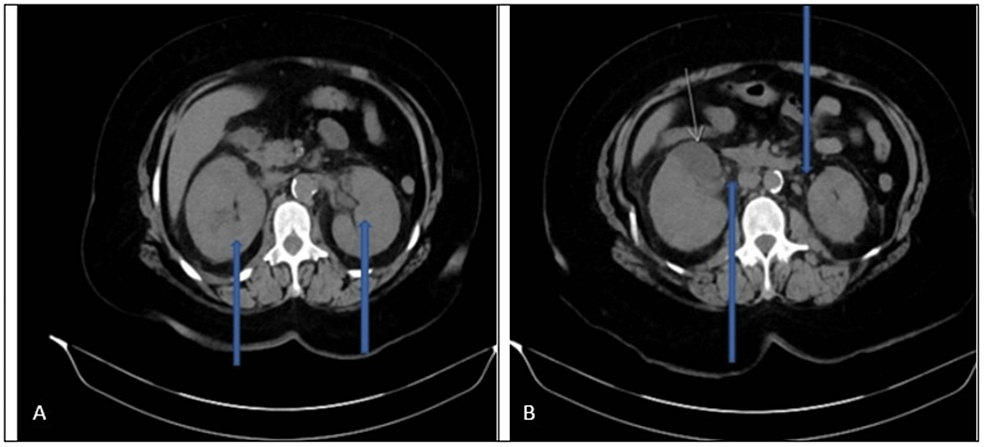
CT abdomen without contrast showing bilateral enlarged kidneys as shown by the blue arrows (A). Additionally bilateral perinephric fat stranding (blue arrows) with wedge-shaped low density in the anterior pole of the right kidney as shown by the yellow arrow (B) consistent with radiologic findings of bilateral acute pyelonephritis.
